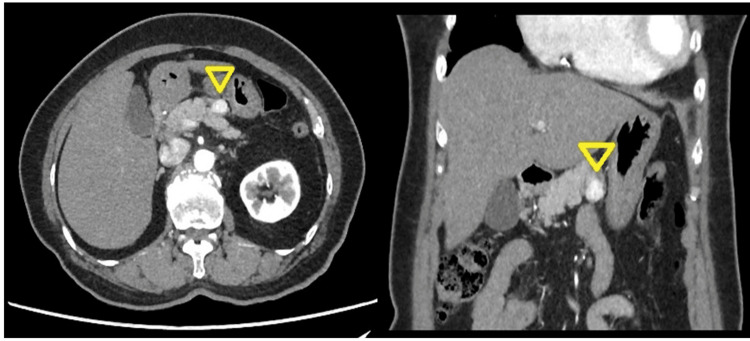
CT image of the tumor (A) Axial view of contrast-enhanced CT reveals a hypervascular lesion (arrowhead) situated in the body of the pancreas. (B) Coronal view of contrast-enhanced CT demonstrates the extent of the pancreatic tumor (arrowhead) CT: computed tomography
